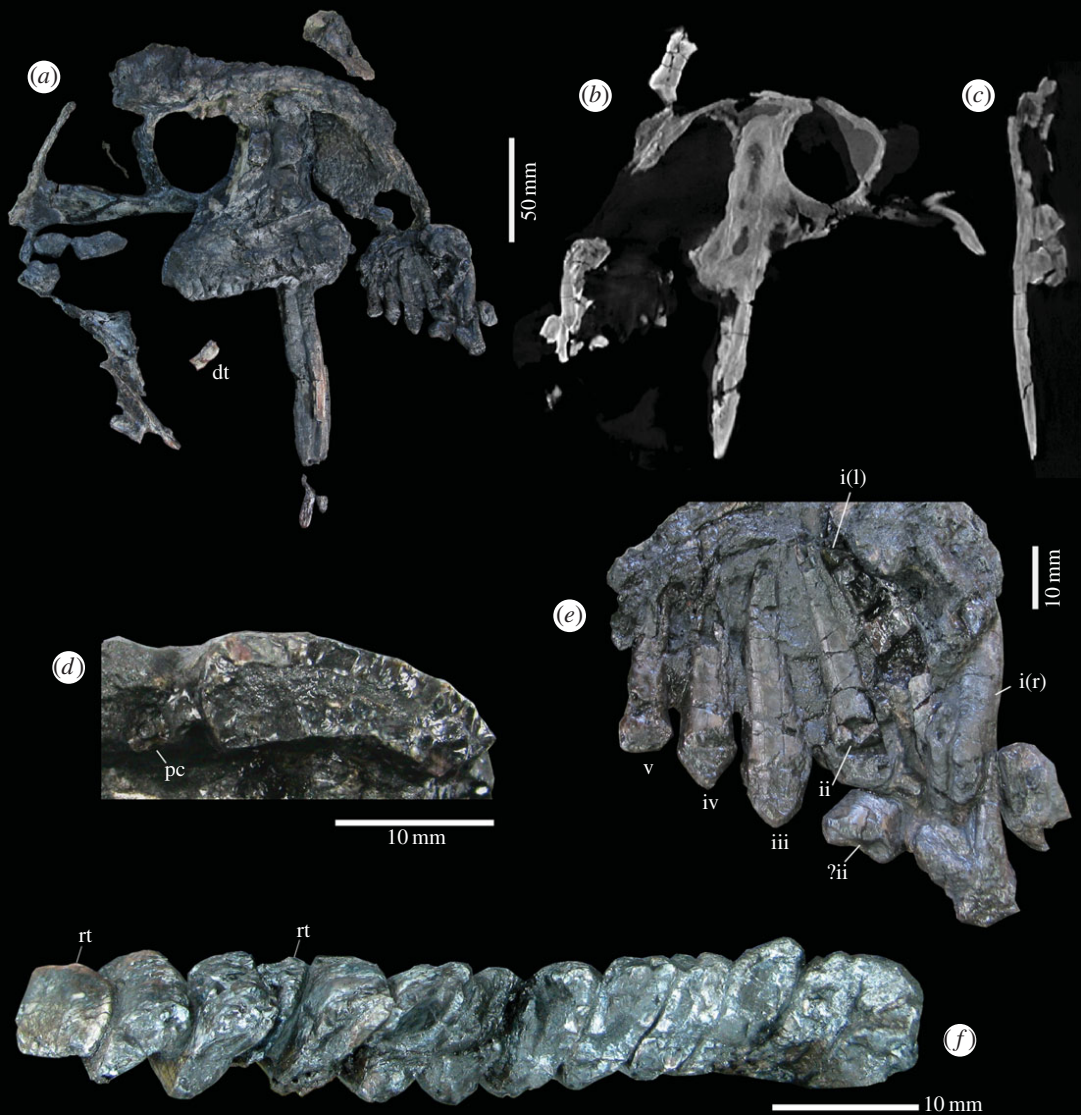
Cranium and dentition of *T. eccentricus*. (*a*) Cranium, left lateral view. (*b*) Cranium, parasagittal section (CT scan), right view. (*c*) Cranium, transversal section (CT scan) at the level of the caniniform, posterior view. (*d*) Ventral view of the base of the left caniniform (exposed due to a fracture) and a precaniniform, anterior to the left. (*e*) Incisiform teeth, medial view. (*f*) Left palatal teeth, occlusal view, anterior to the right. i–iv, tooth positions; dt, disarticulated teeth; l, left; pc, precaniniform; r, right; rt, replacement tooth.
